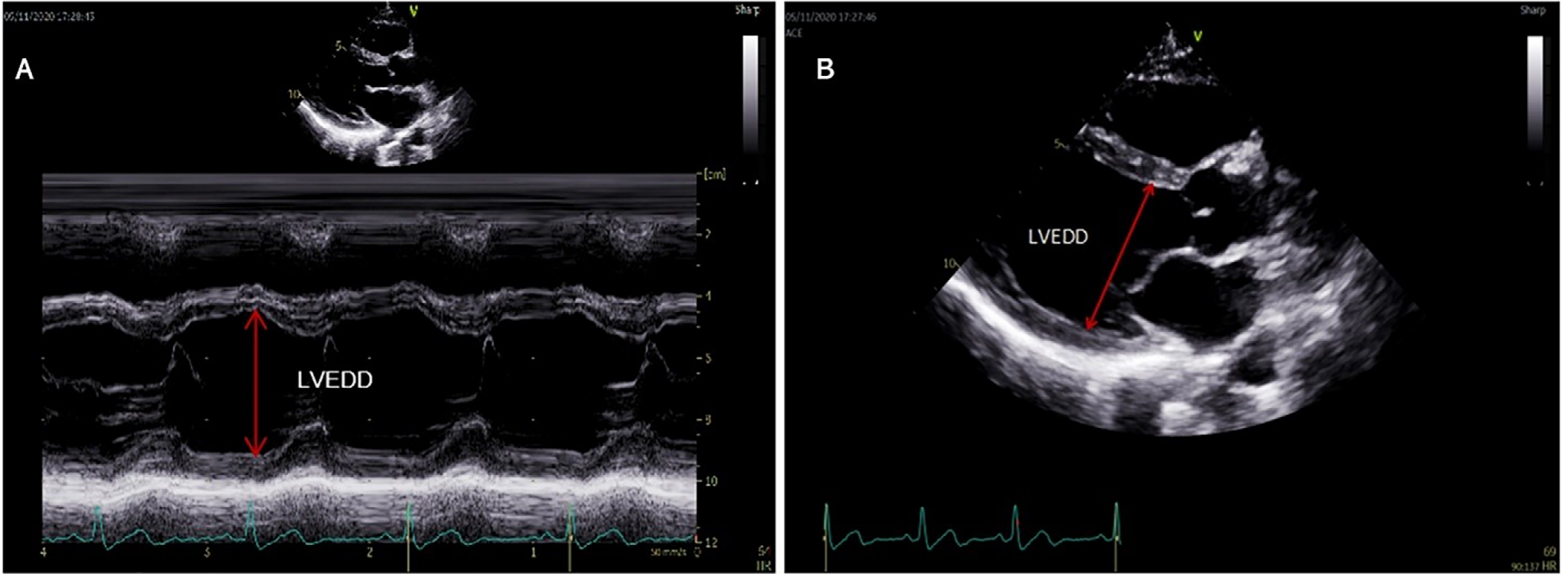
Measurement of left ventricular end‐diastolic diameter (LVEDD). (A) M‐mode echocardiogram showing LVEDD as the maximal internal dimension of the left ventricle during diastole. (B) 2D parasternal long‐axis view demonstrating the same measurement with directional annotation.
